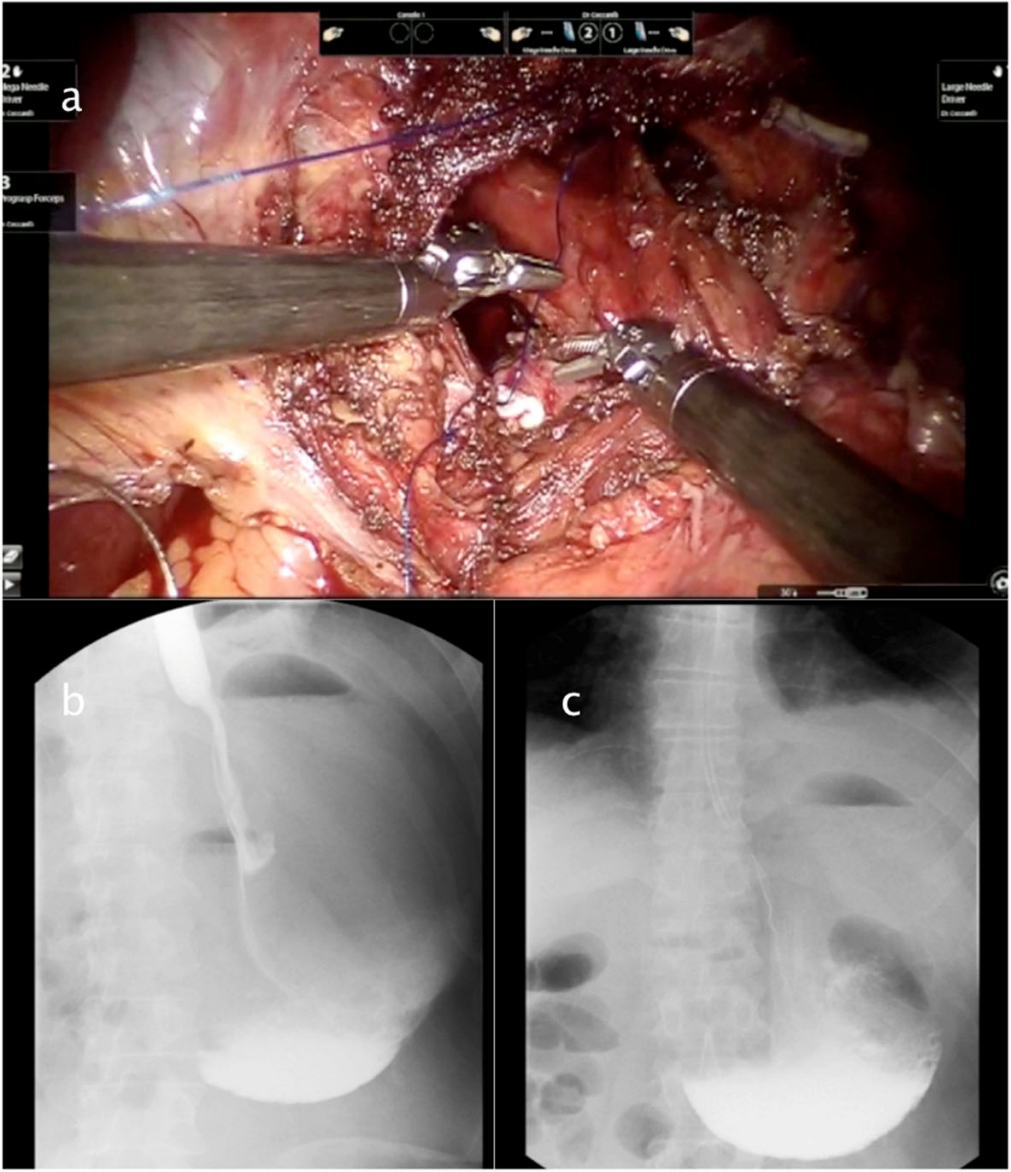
Case 2: Intraoperative images and postoperative X-ray. a Hiatoplasty. b–c A 10 postoperative days X-ray swallow showing a good oesophagogastric transit with antrum stenosis, treated by several pneumatic dilatation after patient discharge from the hospital
